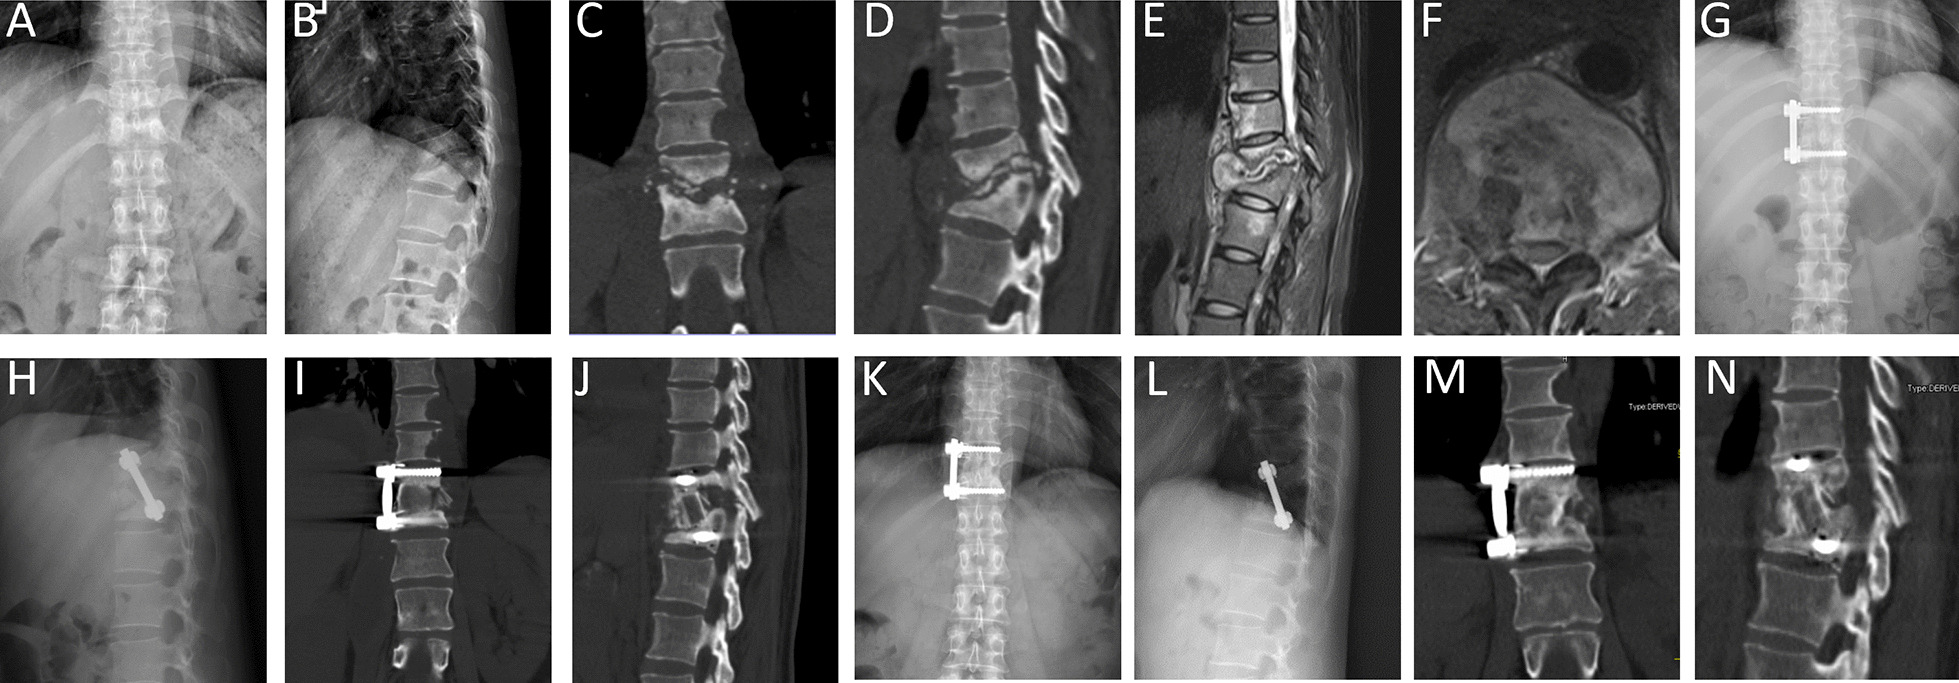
A 45-year-old woman with T11–12 tuberculosis. A, B Preoperative X-ray before surgery demonstrated vertebral bone destruction and kyphosis at T11–12. C–F Preoperative CT and MRI showed T11–12 vertebral bone destruction with paravertebral and intraspinal abscess. G–J Postoperative X-ray and CT of the patient underwent anterolateral debridement and instrumentation at T11–12 with autologous structural iliac bone graft. K–N X-ray and CT at 25 months postoperative showed good bone fusion and spinal alignment without signs of tuberculosis recurrence and hardware failure
